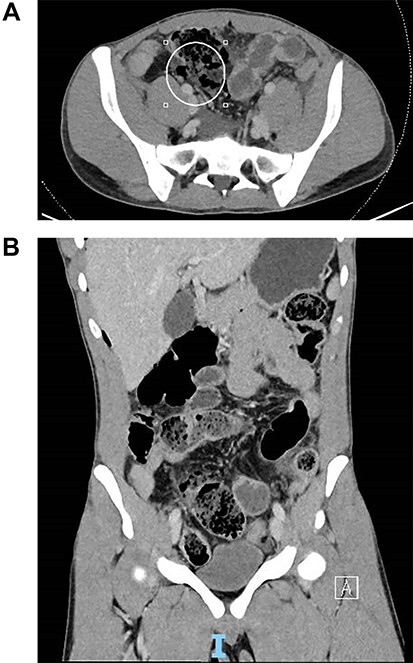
(A) Coronal slice of computed tomography (CT) image of small bowel perforation at distal ileum. (B) Sagittal slice of CT image of small bowel perforation at distal ileum.
